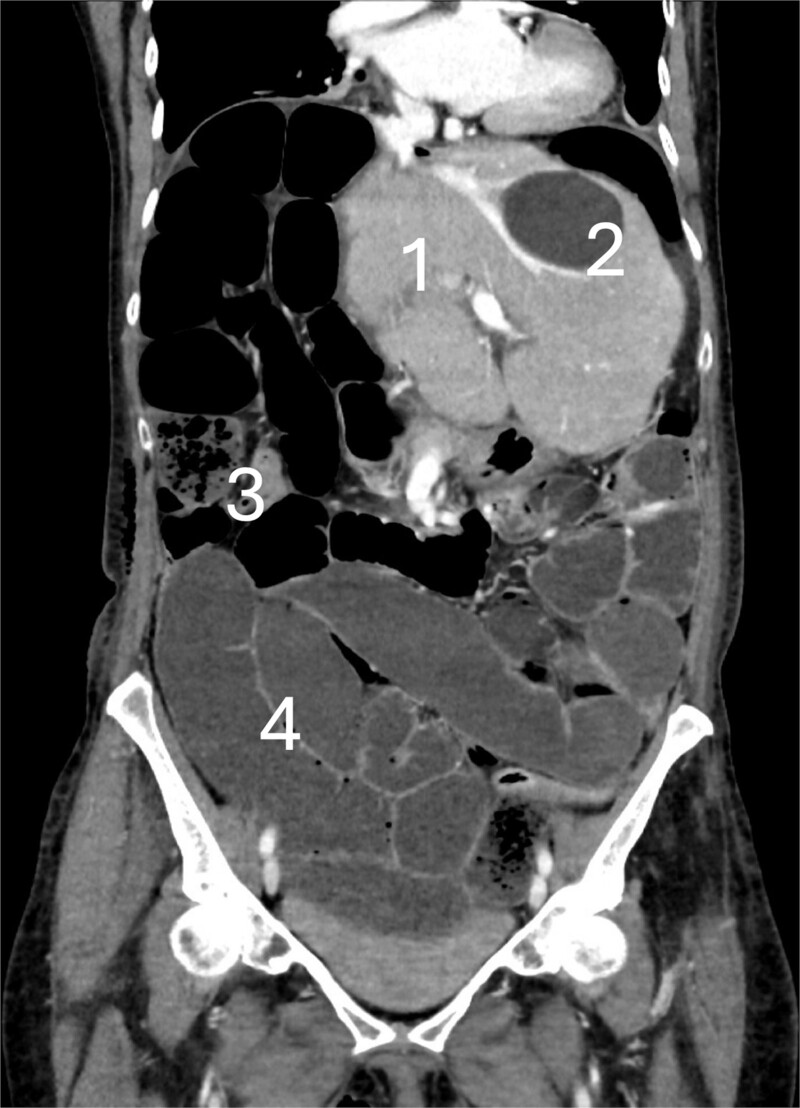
CT scan at the 6th postoperative day after hepatectomy showing cecal volvulus with: (1) Remnant liver, (2) benign liver cyst, (3) small bowel feces sign, and (4) fluid-filled dilated small bowel.
